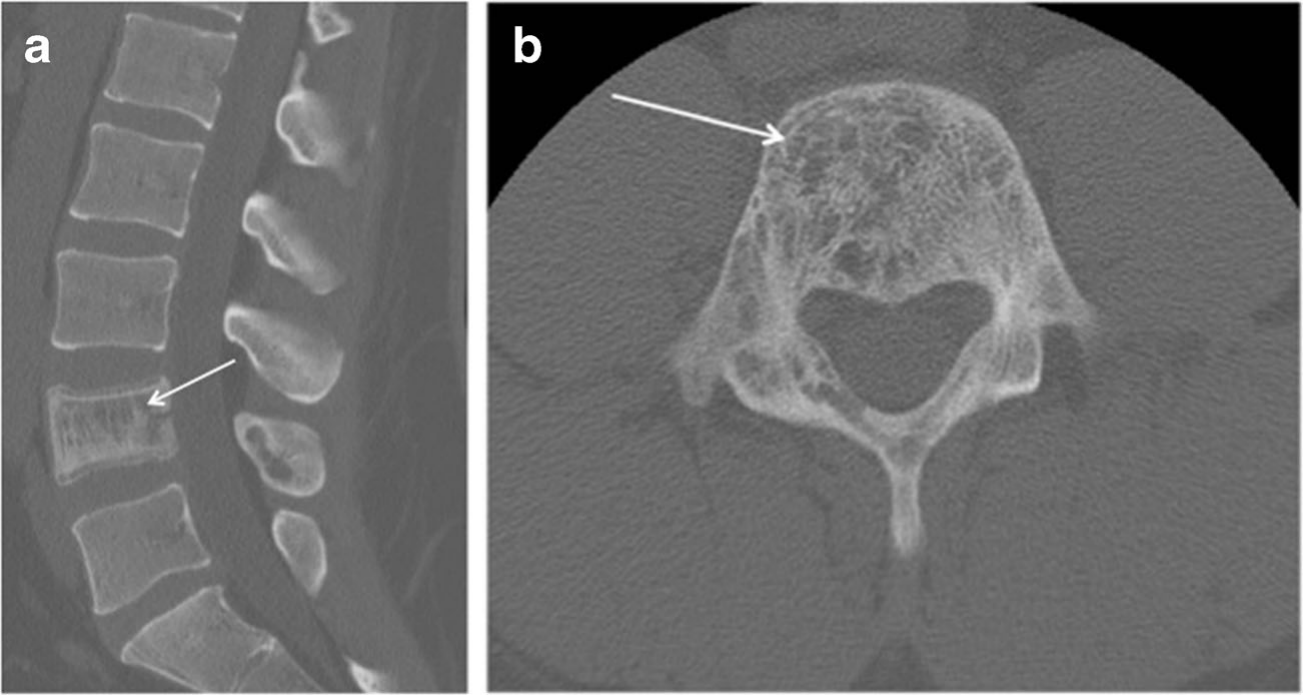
Sagittal CT image (a) shows the classical striated appearance of a haemangioma in the L4 vertebral body. Axial CT image (b) shows the spiculated, spotted appearance of the lesion in cross-section
